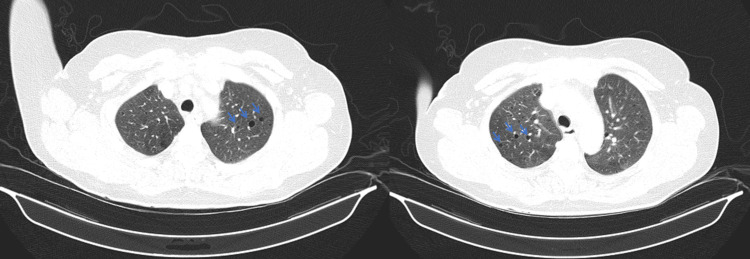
Thoracic CT. Multiple cystic images in the lung parenchyma (blue arrow). CT: computed tomography
